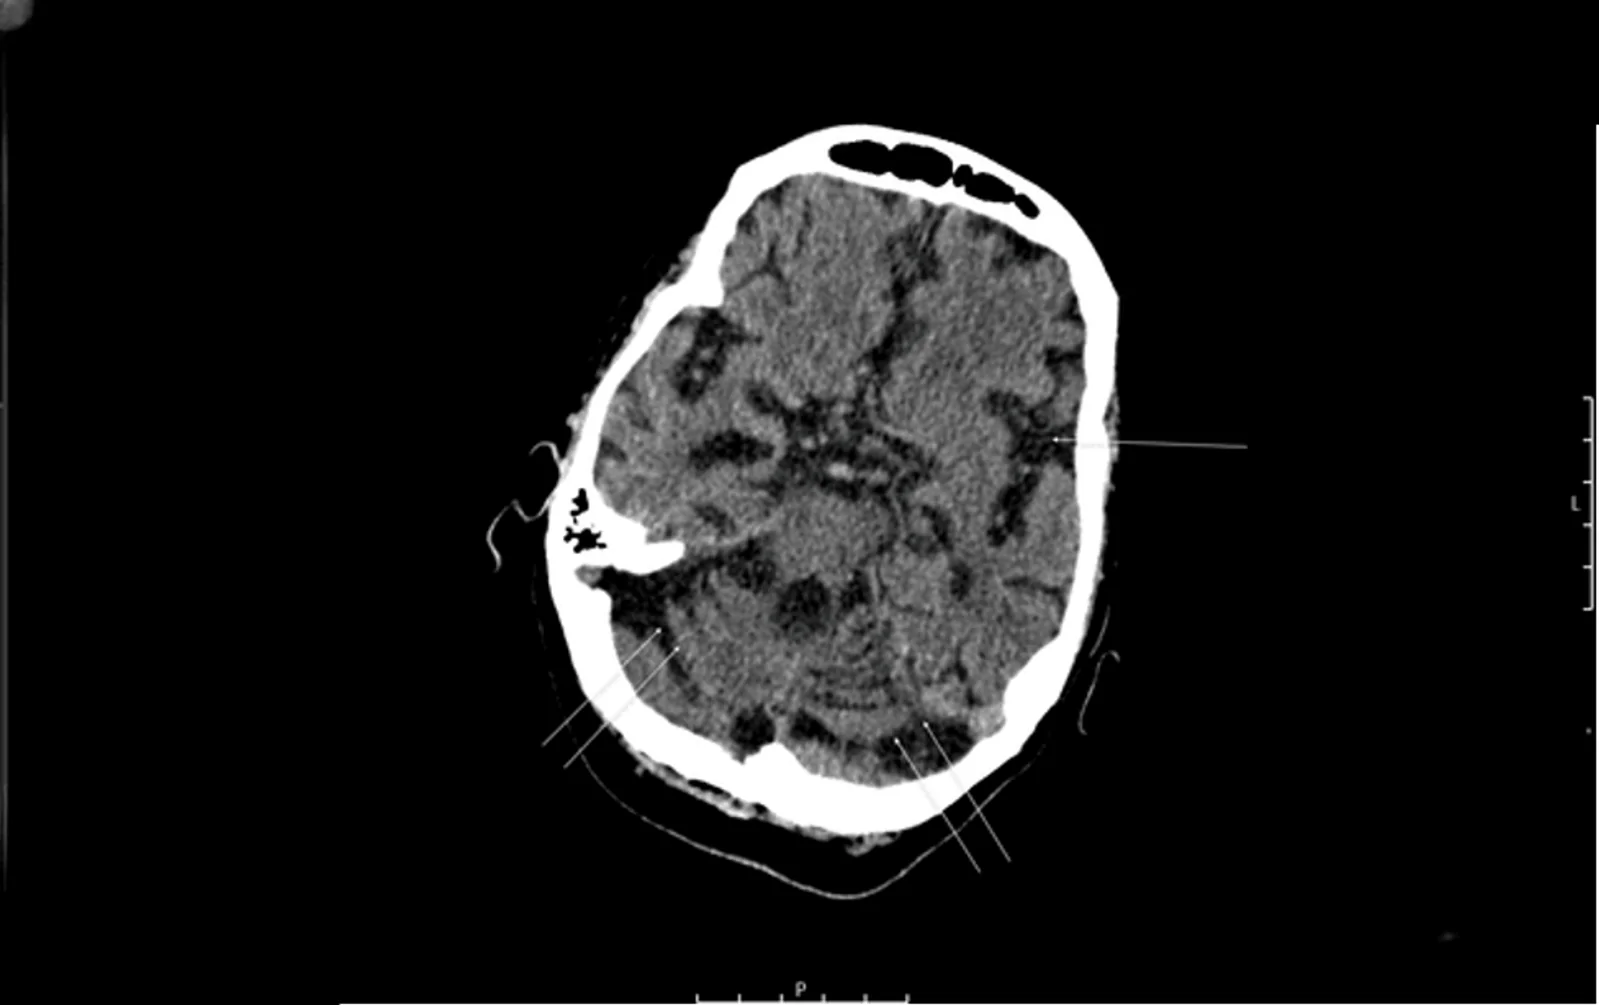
CT of the head performed at the age of 72. White arrows show moderate neuroparenchymal atrophy, more prominent in the cerebellum.
